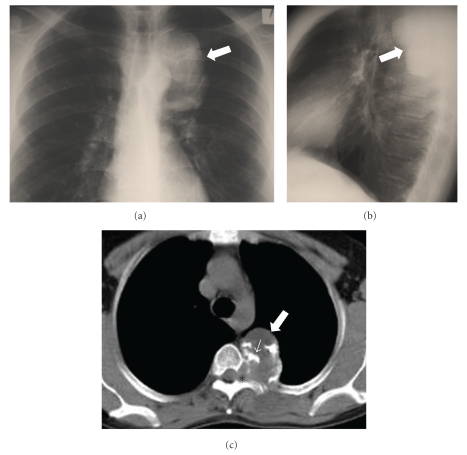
A 32-year-old man with chondrosarcoma. The posteroanterior (a) and lateral (b) chest radiographs, show a well-defined radiopaque lesion in the left posterior paraspinal location (arrows). (c) The axial MDCT image demonstrates a soft-tissue mass (arrow) with amorphous “rings and arcs” calcified matrix (thin arrow) and adjacent neural foramina widening (asterisk).
